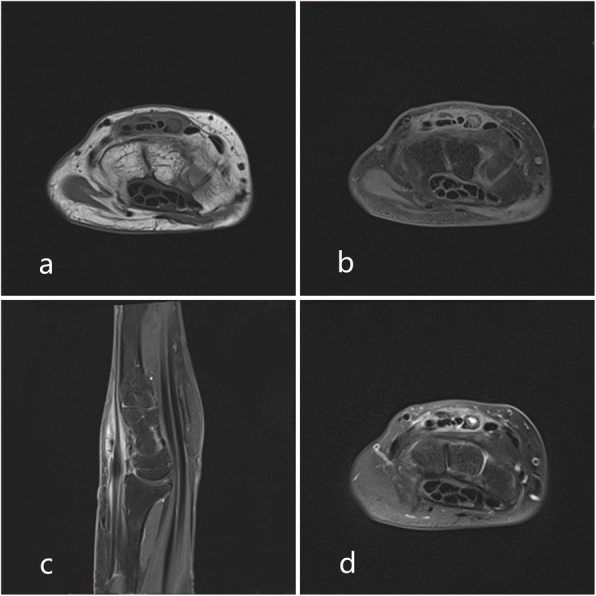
MRI (a,b,c) reveals a 18 × 6× 5 mm spindle-like mass,encircles the extensor indicis proprius tendon in her left dorsal wrist.The lesion shows iso-indensity on T1 image (1a),while it shows hyperintense signal on T1WI with fat suppression(b: axial plane; c: sagittal plane) and T2WI with fat suppression (d).On T2WI with fat suppression (d), we can see the mass (shows hyperintense signal in the center and iso-indensity in the peripheral) attached to the EIP tendon(shows hypointense signal)
